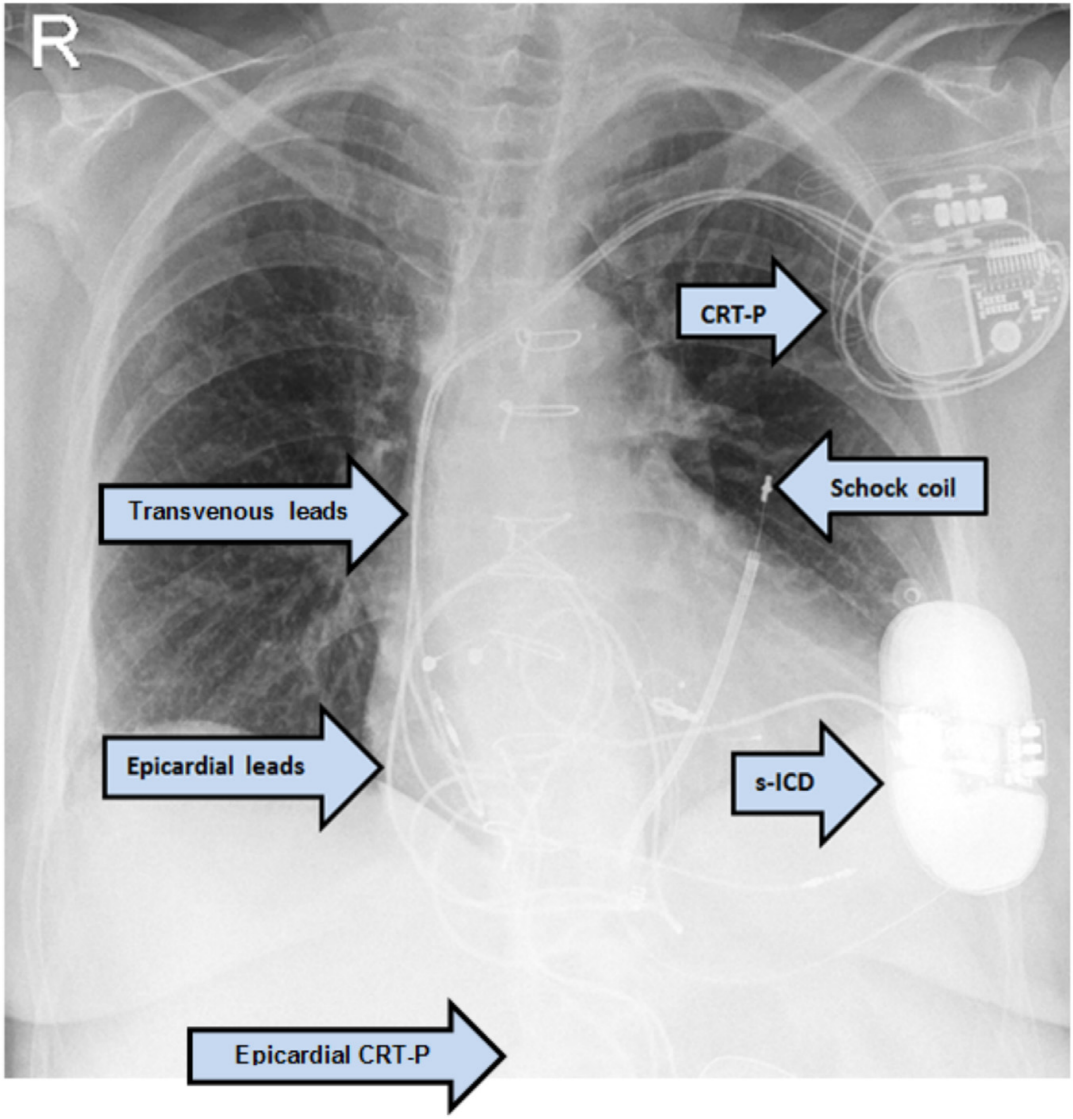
The patient's most recent chest radiograph, posteroanterior view.
